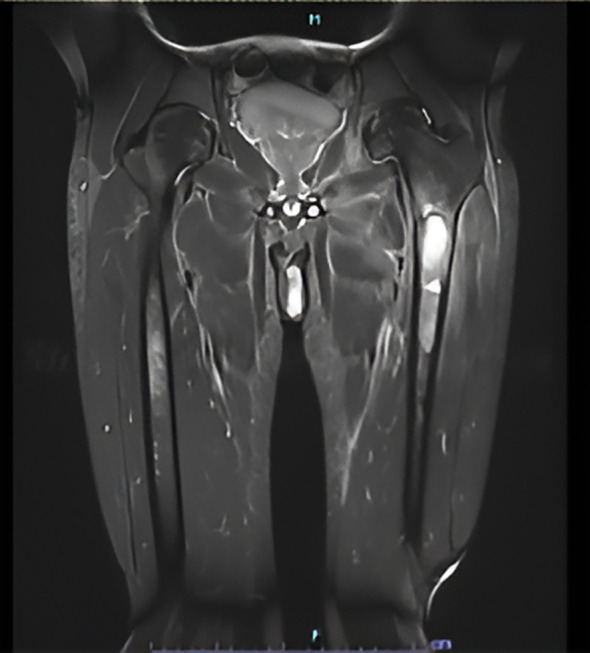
MRI Pelvis coronal (arrow pointing to metastatic lesion in left femur).
